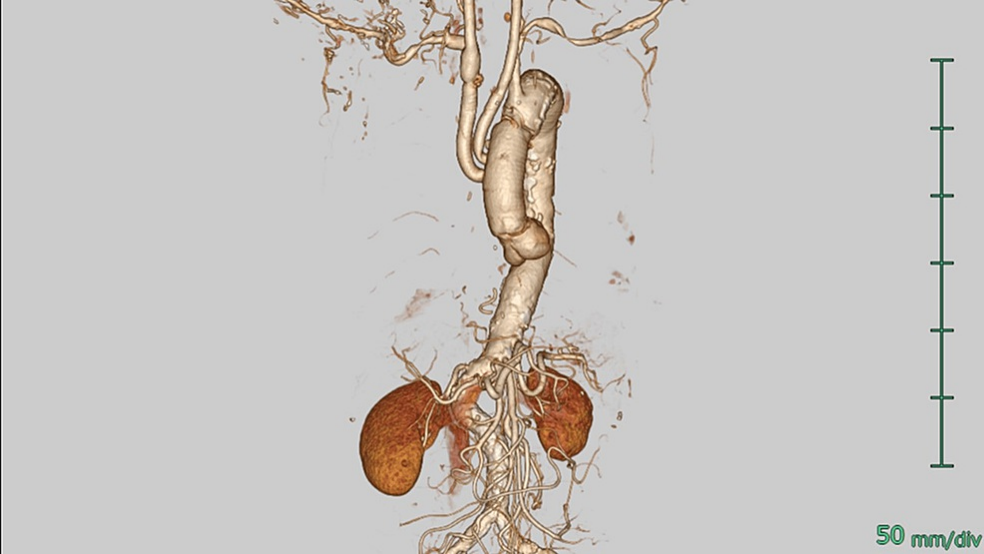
Postoperative CT partial arch replacement with dilated innominate artery resection.
